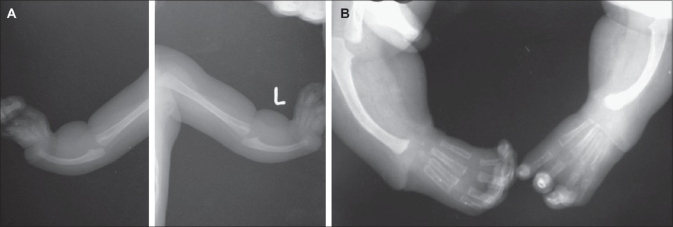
X-ray of bilateral Club hand (A) before and (B) after surgery
